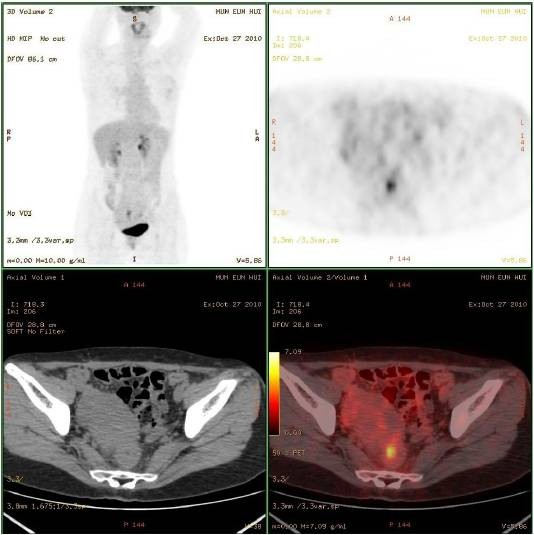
FDG-PET showed hypermetabolism in the rectum. The mean standardized uptake value calculated by FDG-PET for rectal tumor was 7.6.
